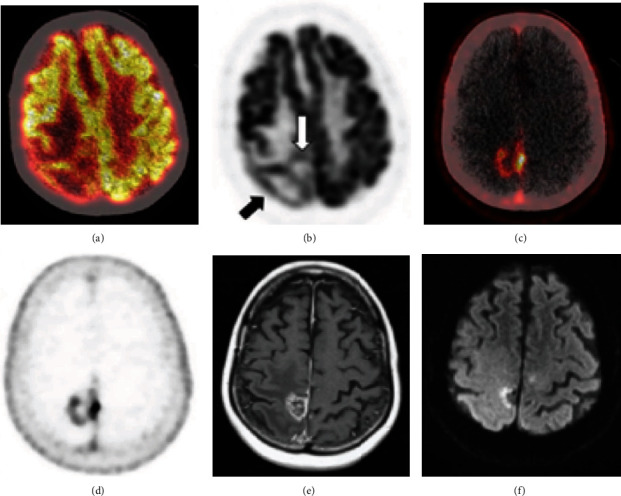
^18^F-FDG and ^18^F-PSMA PET MRI imaging of representative breast cancer brain metastasis. (a) ^18^F-FDG PET/CT. (b) ^18^F-FDG PET. (c) ^18^F-PSMA-1007 PET/CT. (d) ^18^F-PSMA-1007 PET. (e) T1-weighted MRI. (f) DWI MRI. Reproduced with permission from Figure 1 in Marafi et al. [68].
